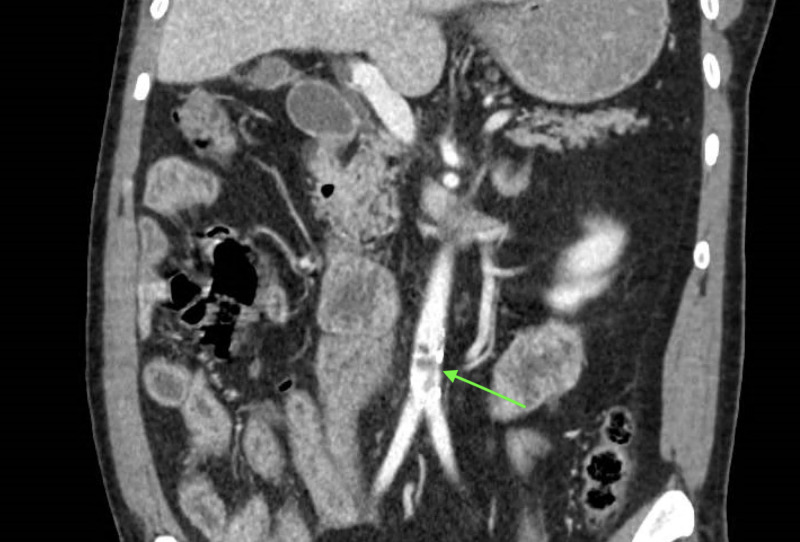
Abdominal CT scan with contrast Coronal view: showing irregular noncalcified thrombus in the distal infrarenal segment of the vessel significantly narrowing the effective lumen of the abdominal aorta CT: computed tomography
